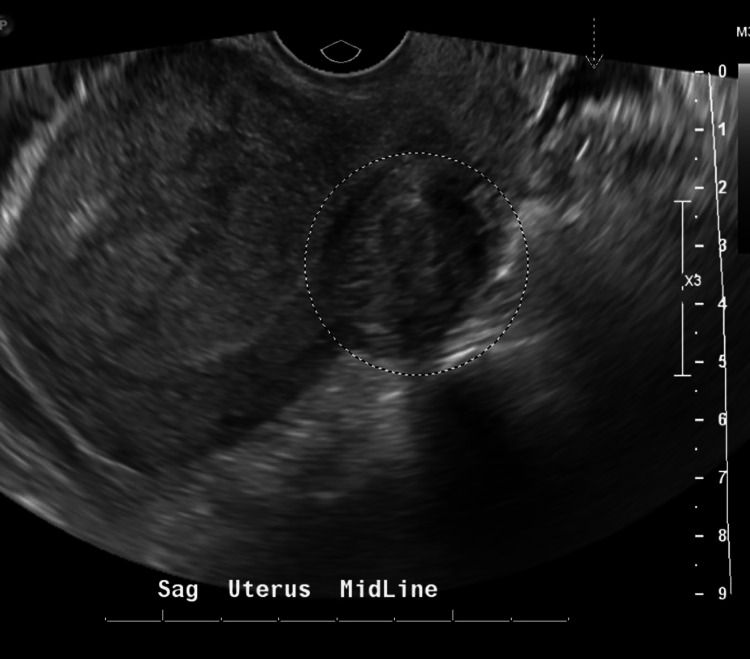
Transvaginal pelvic ultrasound image Transvaginal pelvic ultrasound showing fibroids (circle) with only trace free fluid in the pelvis (arrow).
